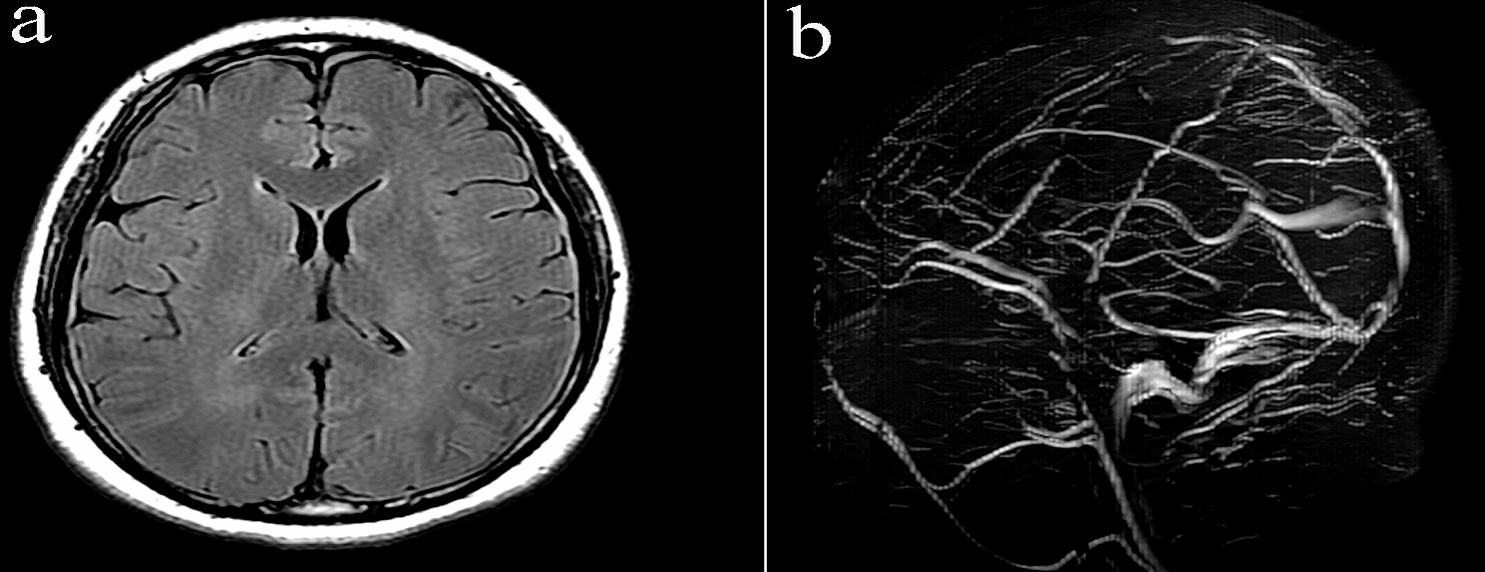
Craniocerebral MRI scan showed narrowing or disappearance of the sulci, thickening and enhancement of the dura (a). The brain MRV showed that the swollen left parietal cortex, venous thrombosis (part of the superior sagittal sinus and local draining vein), possible venous infarction of the left parietal lobe, and the indistinct superior sagittal sinus (b). MRI: Magnetic Resonance Imaging; MRV: Magnetic Resonance Venography
